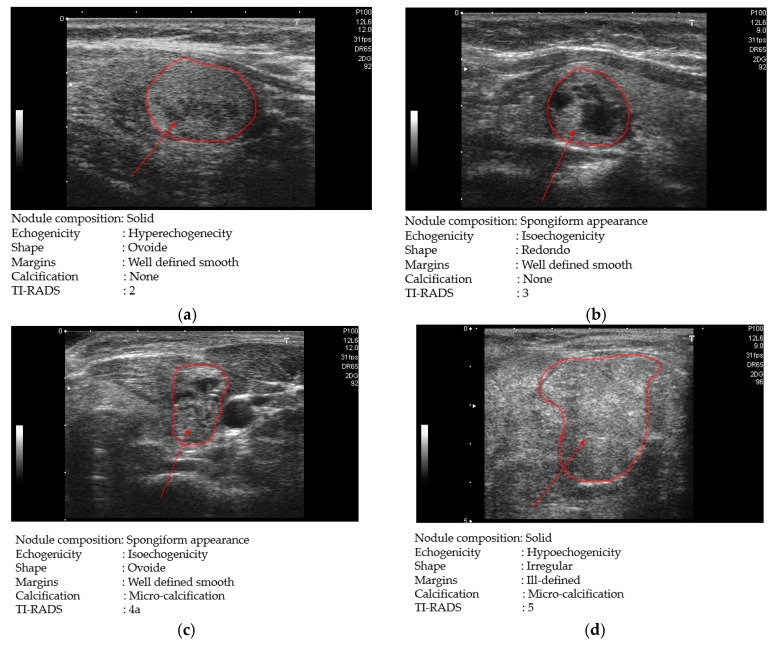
Examples of ultrasound thyroid images in TDID dataset: (a) a benign case image with thyroid imaging reporting and data system (TI-RADS) score of 2; (b) a benign case image with TI-RADS score of 3; (c) a malign case image with TI-RADS score of 4; and (d) a malign case image with TI-RADS score of 5.
